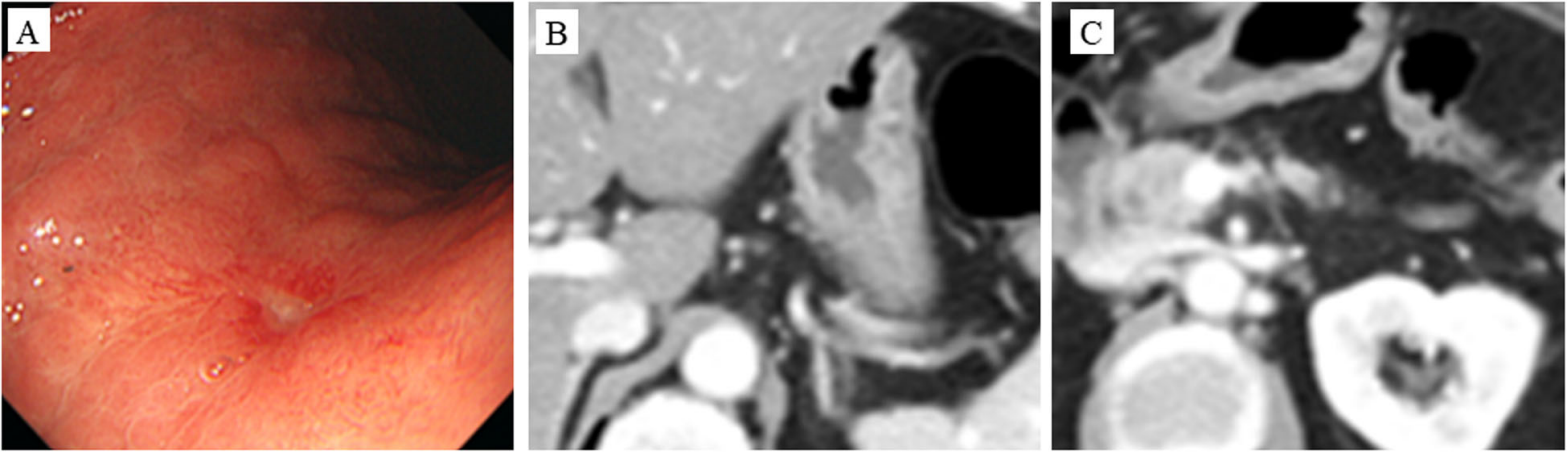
Esophagogastroduodenoscopy (EGD) and computed tomography (CT) findings after chemotherapy. a EGD after chemotherapy detected a scar lesion instead of a tumor. Abdominal CT after the five courses of S-1, oxaliplatin, and trastuzumab revealed regression of the regional lymph nodes (b) and para-aortic lymph nodes (c)
